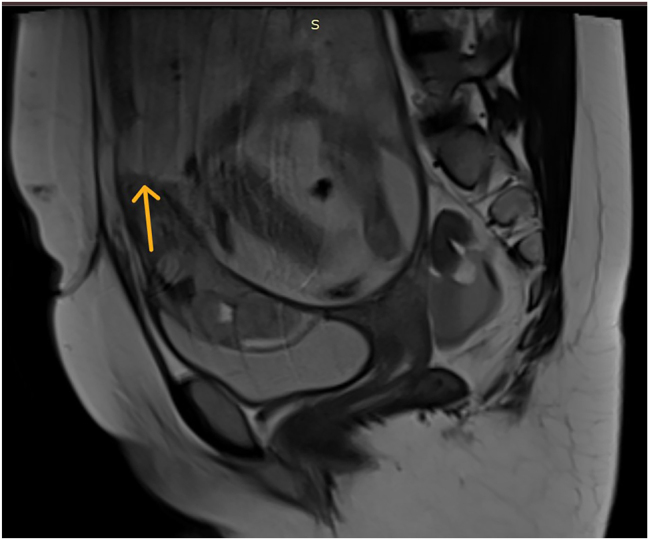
T1-weighted sagittal MRI showing hemorrhagic changes at the uterine rupture site.
